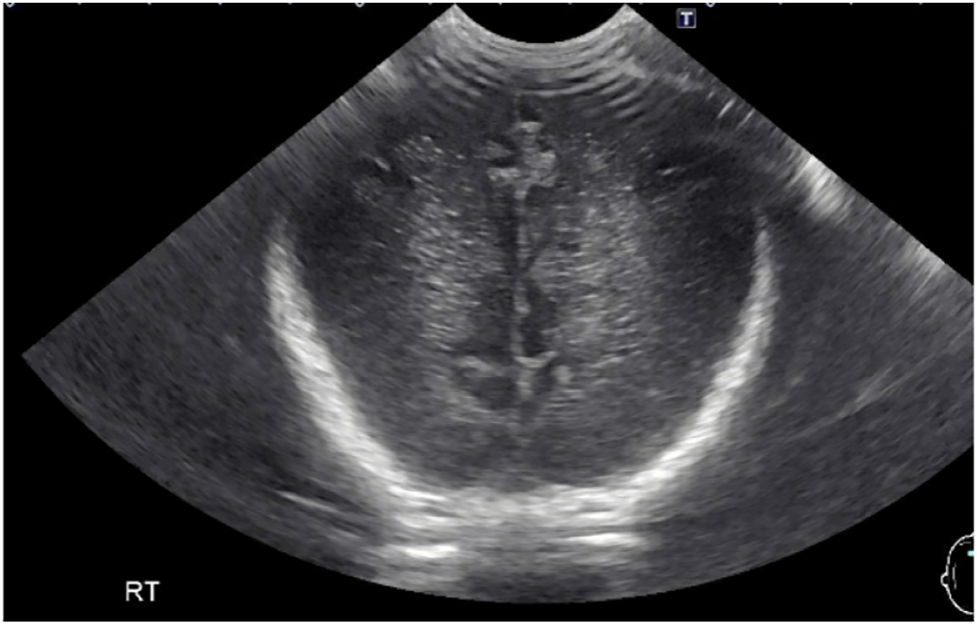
Cranial ultrasound revealed hemorrhage in the caudothalamic groove along with intraventricular extension bilaterally. There was mild prominence of the right lateral ventricle. Overall findings are compatible with right grade 3 hemorrhage and left grade 2 hemorrhage.
